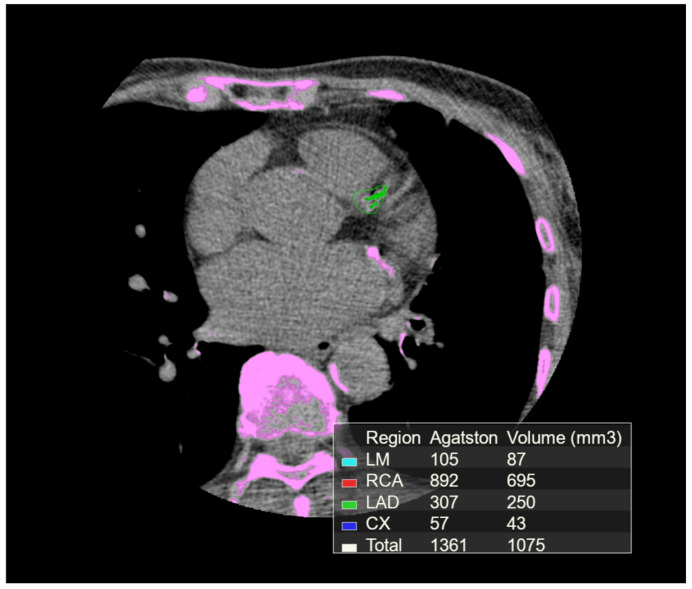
CT-based coronary calcium scoring. Axial non-contrast cardiac CT image showing automated detection and color-coded segmentation of calcified plaques in the coronary arteries, with corresponding Agatston scores and calcium volumes reported for each vessel and for the total coronary calcium burden.
